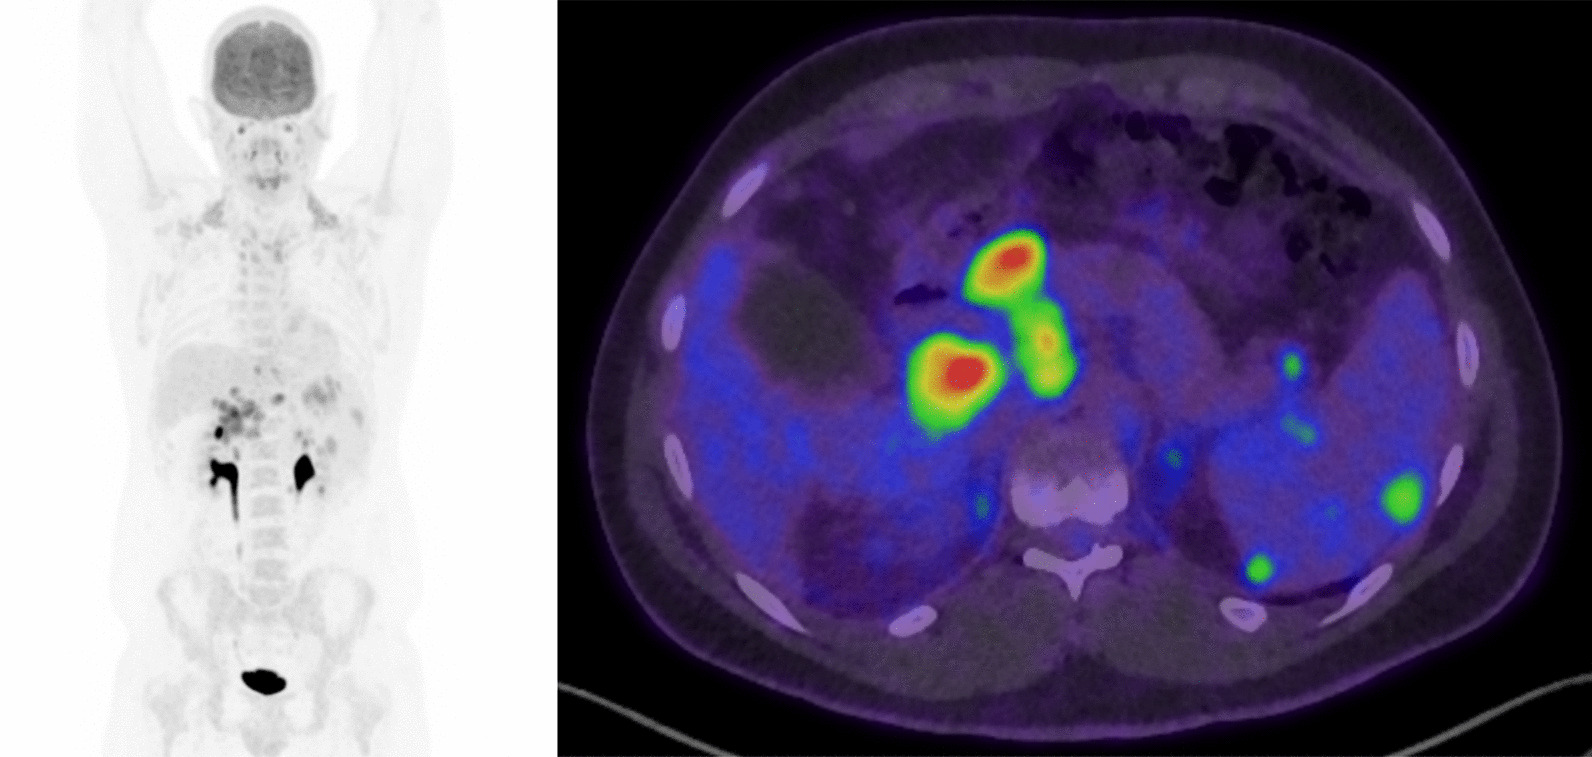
Fluorodeoxyglucose positron emission tomography scan. The fluorodeoxyglucose positron emission tomography scan shows abnormal FDG uptake primarily in the spleen, porta hepatis, and portocaval and paraaortic nodes
